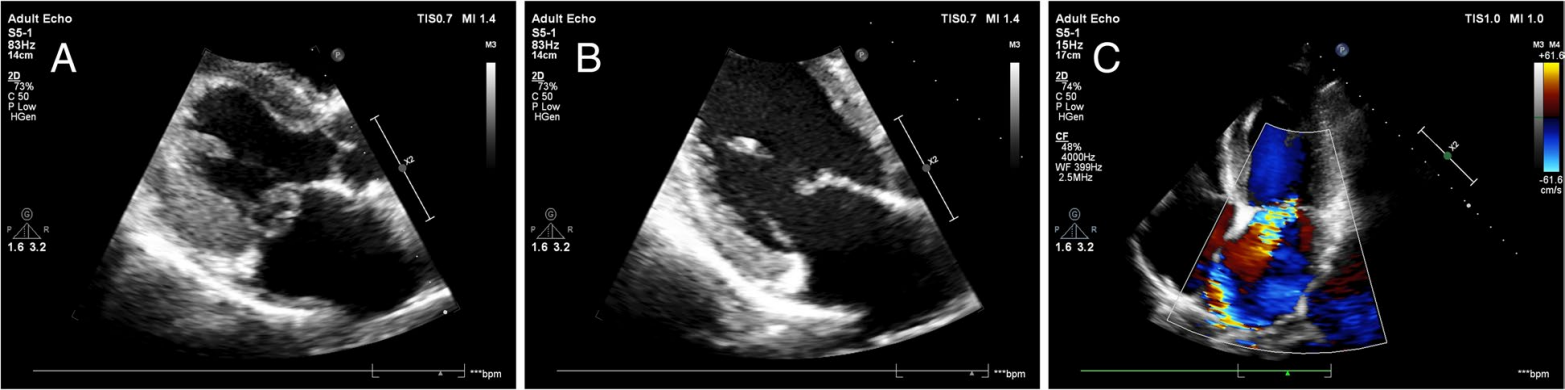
Echocardiogram. A showed mitral valve prolapse. B showed thickened, roughened mitral valve leaflets. C showed severe mitral regurgitation
